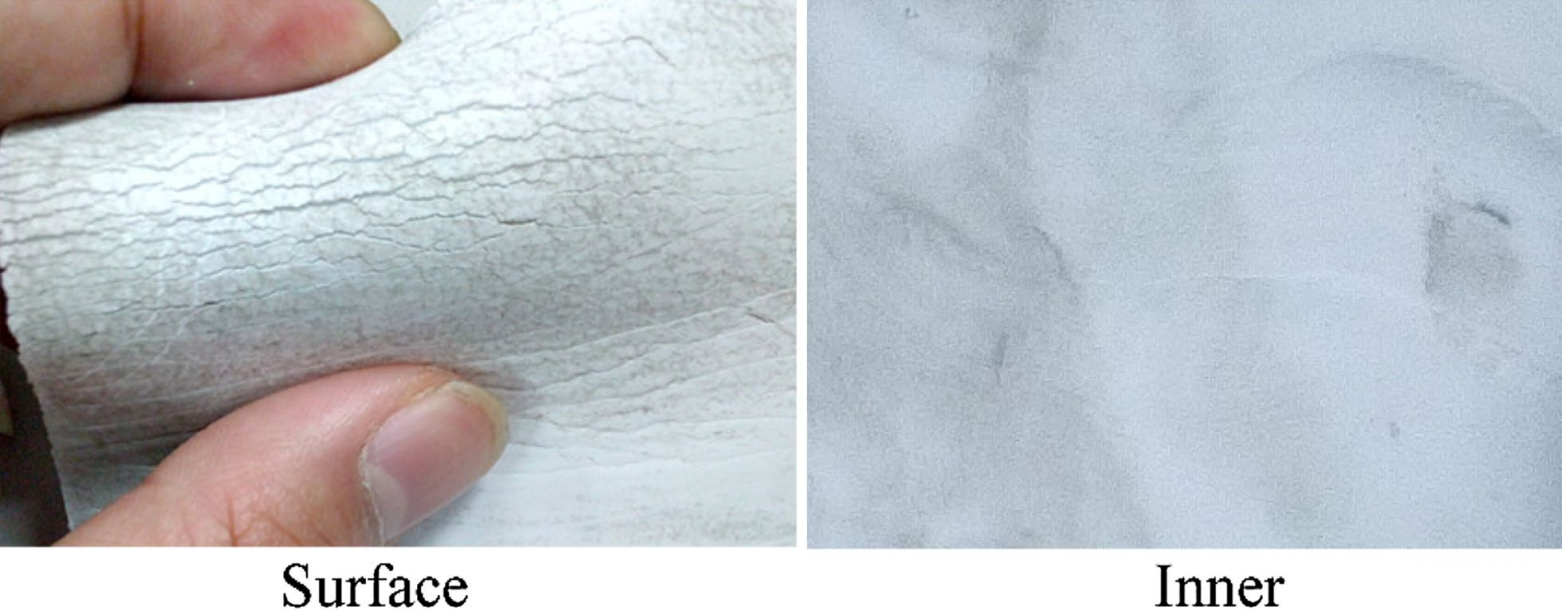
Cracking on the surface part (*left*) and intact inner part (*right*) of the CT insulation bushing
